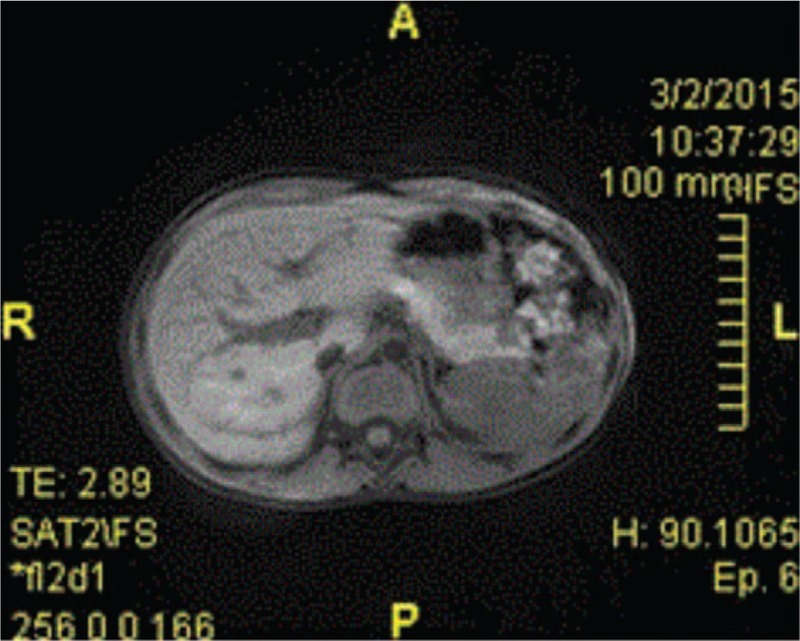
MRI: high signal focal area of 1 cm in diameter in the tail of the pancreas. MRI = magnetic resonance imaging.
